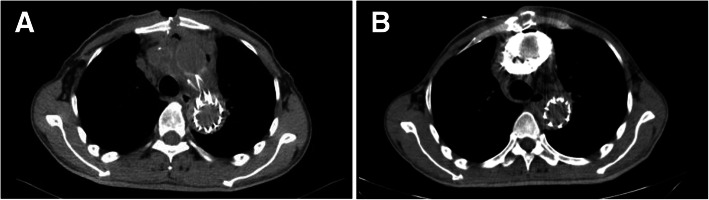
a Computed tomography (CT) shows soft tissue and ectopic gas surrounding the ascending aortic graft having a diameter of 14 mm. b CT scan showing a large amount of contrast agent wrapped around the replacement ascending aorta graft with a small amount of gas
